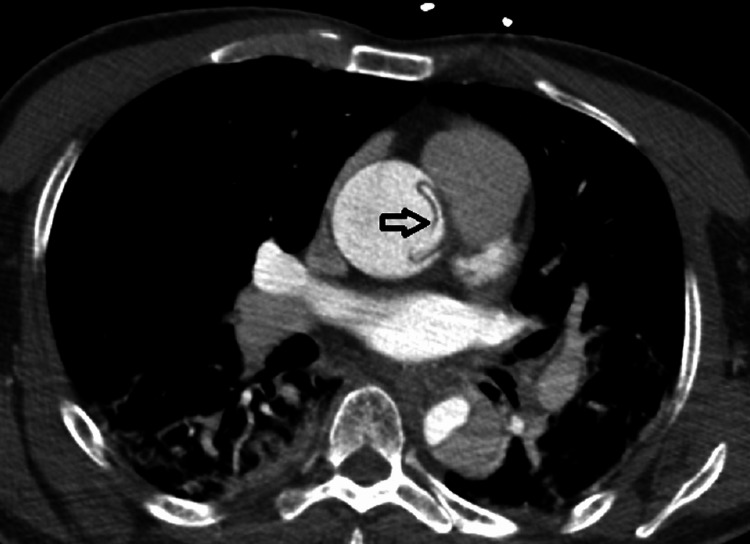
Axial CT angiography image of the thoracic region showing the dissection flap (black arrows) in the descending aorta.
